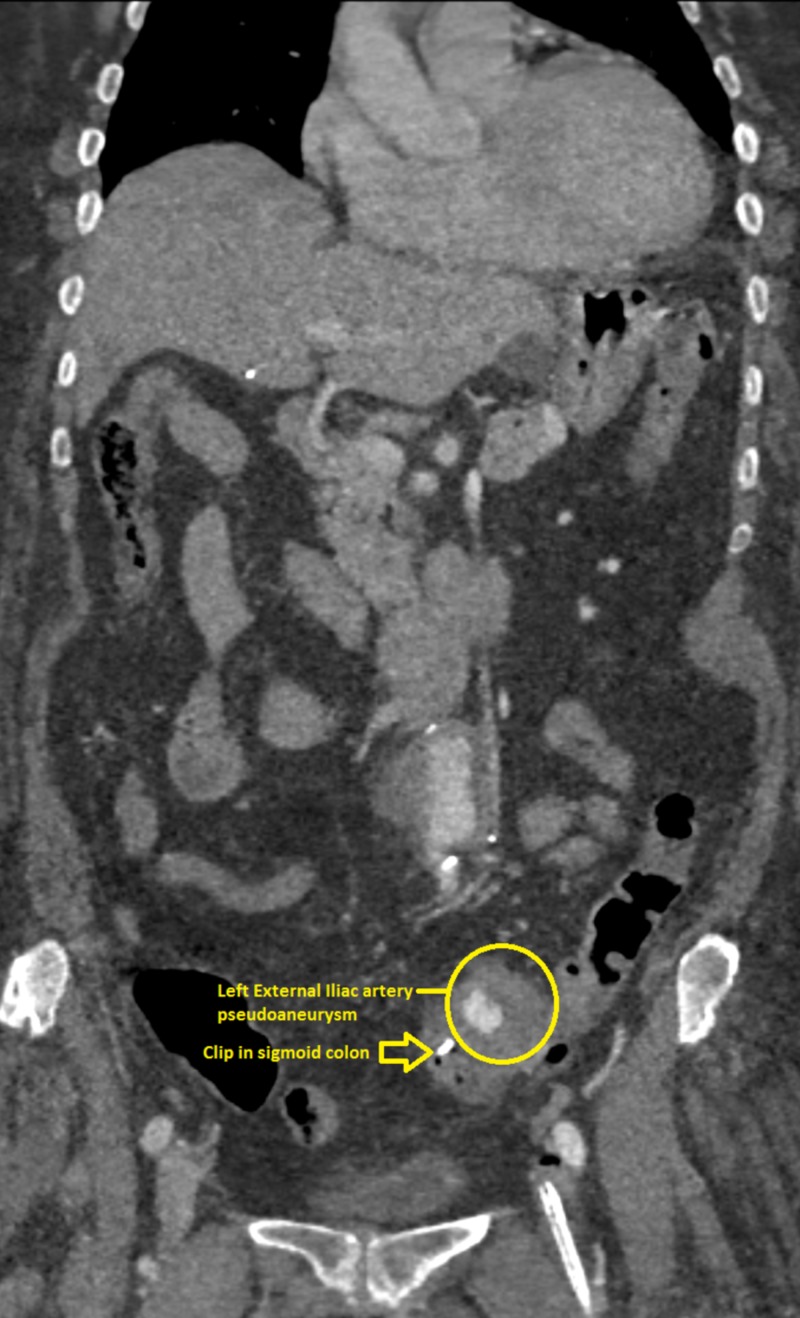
Coronal contrast-enhanced computed tomography (CT) image demonstrates endoscopically placed clip in sigmoid colon adjacent to the partially thrombosed left external iliac artery pseudoaneurysm
